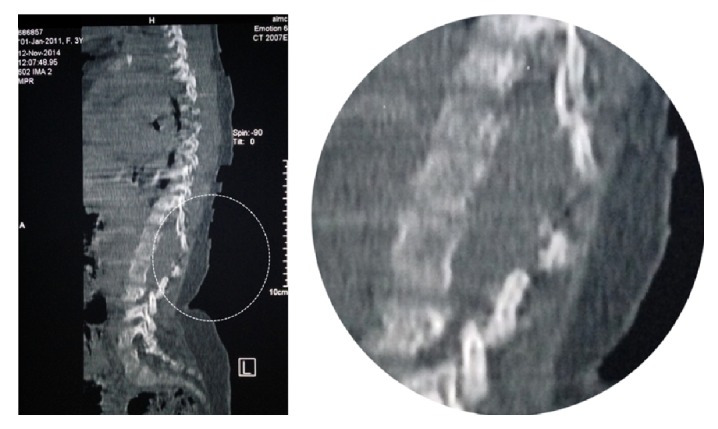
A sagittal CT section demonstrating a multiple level spinal dysraphism and deformity.
